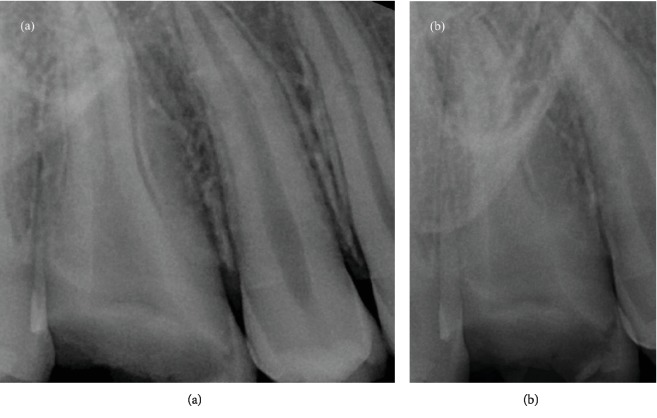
(a) Preoperative orthoradial X-ray; (b) preoperative distal angulated X-ray.
